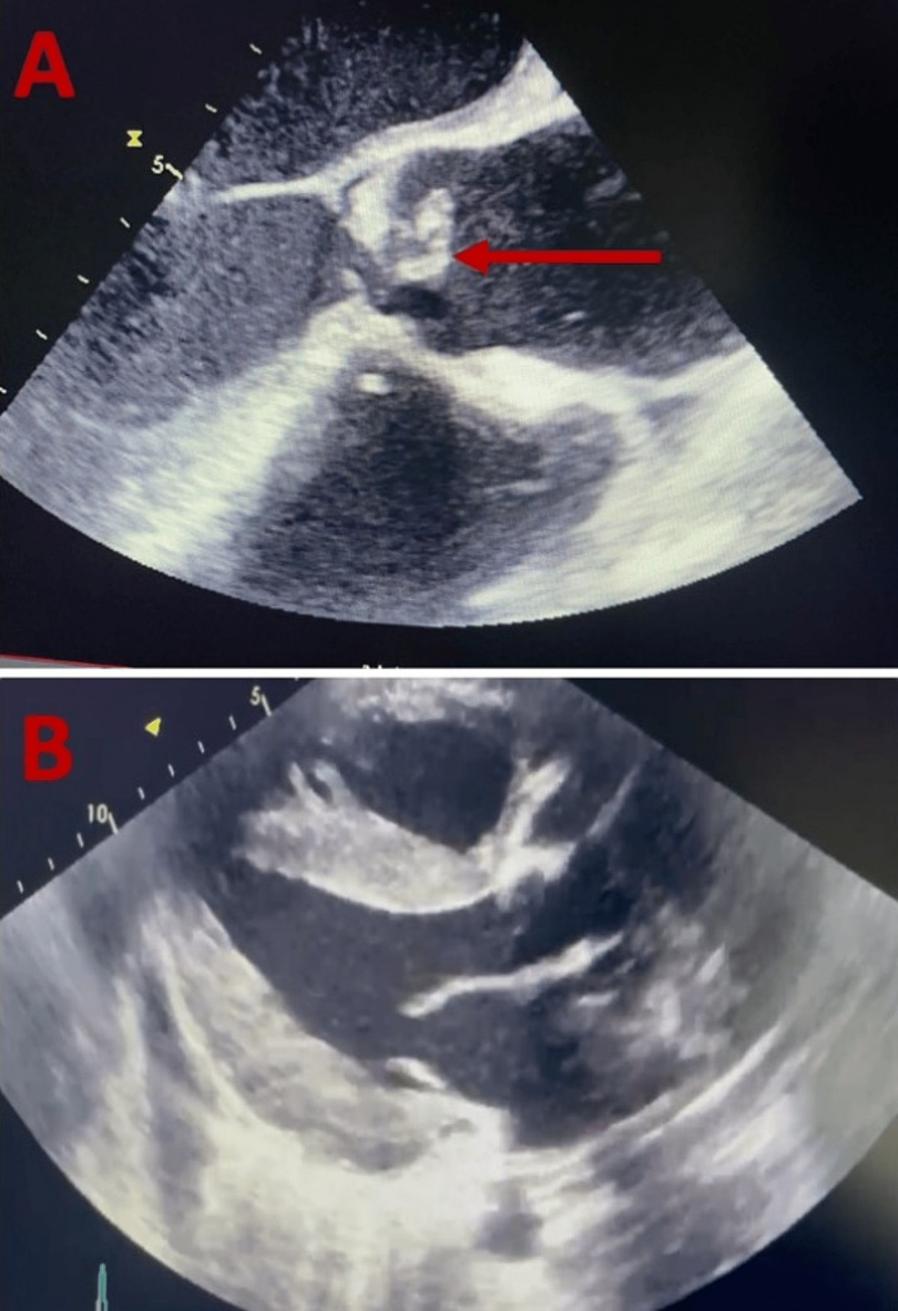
Diagnostic imaging (echocardiography) (A) Parasternal short-axis view with the arrow pointing to a mass attached to the aortic valve (red arrow).​ (B) Postoperative short-axis view showing the absence of the aortic valve mass.
